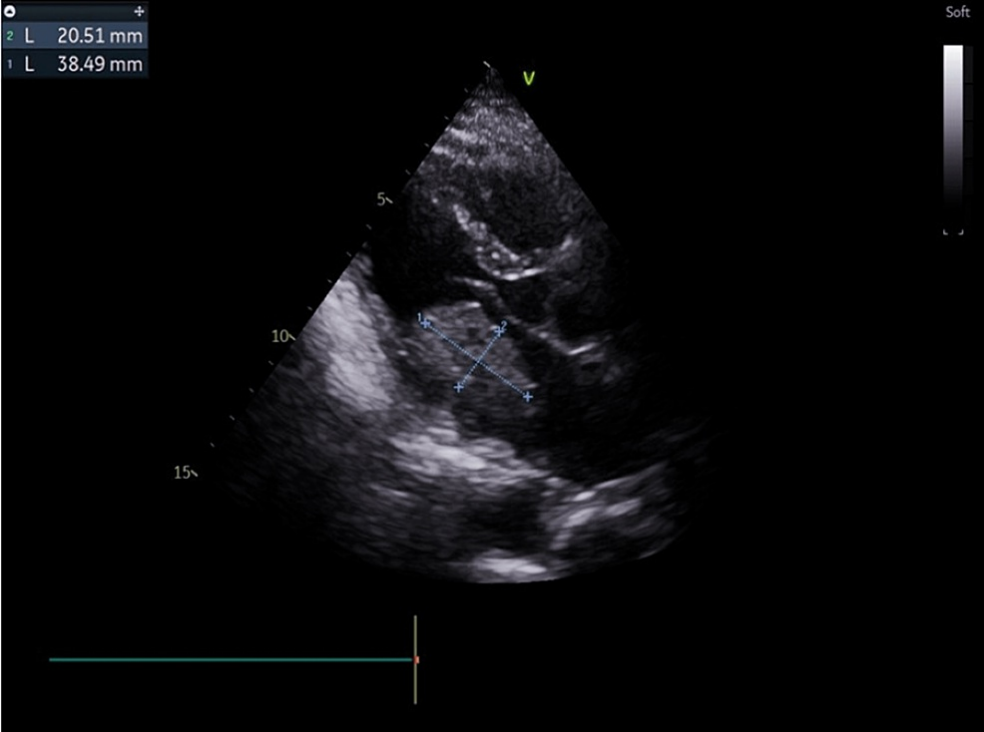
Echocardiographic image obtained in the parasternal long-axis view, showing the myxoma attached to the posterior leaflet of the mitral valve
